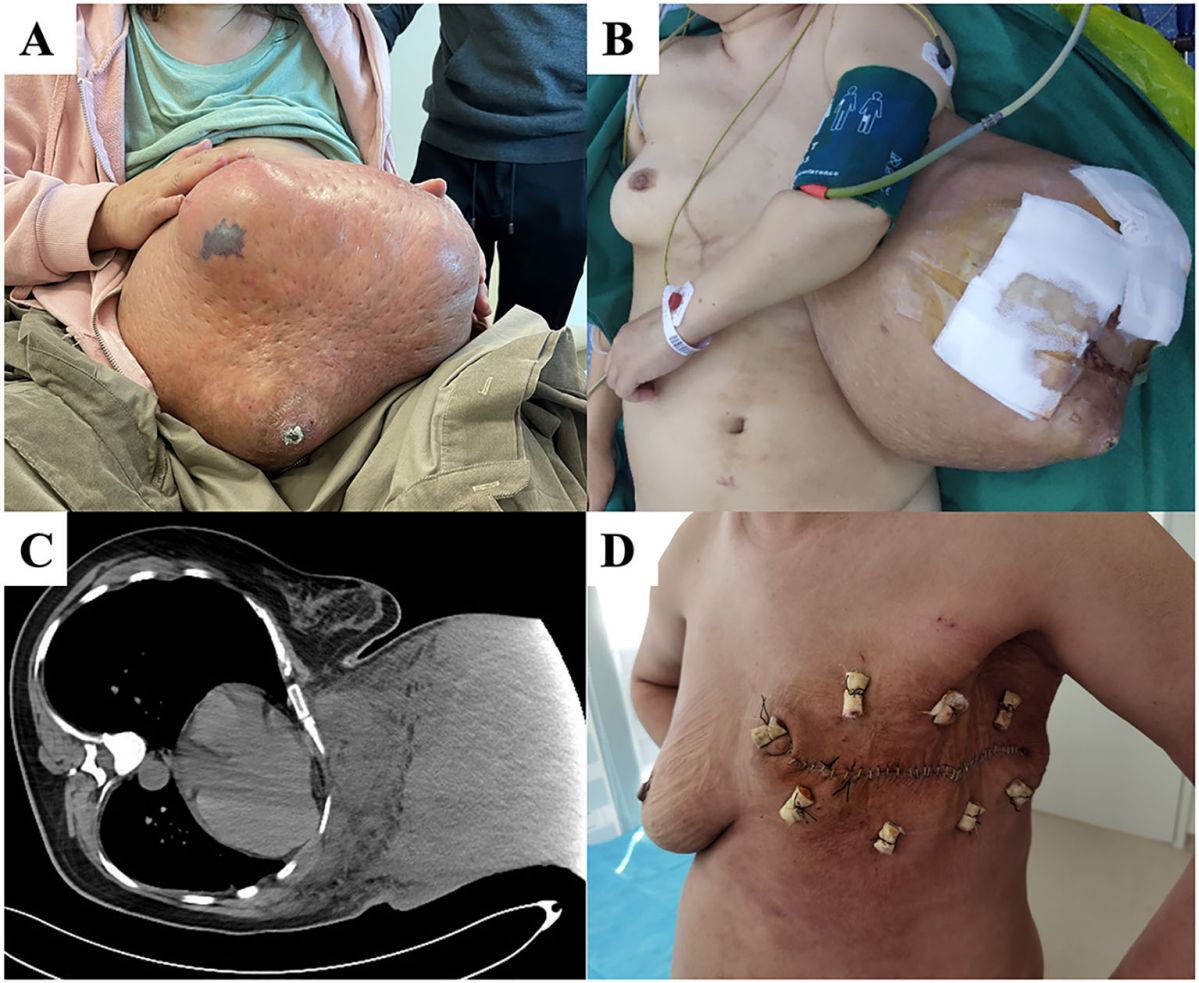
(A) At the time of the first visit, the patient was unable to walk and could not sleep flat on her back due to a huge left breast mass. (B) After general anesthesia, the patient was placed in a flat position and the local skin breakdown was covered with gauze. (C) Chest computed tomography (CT) showing tight adhesion of the mass to the chest wall with localized invasion. (D) The patient’s wound healed well 14 days after surgery.
